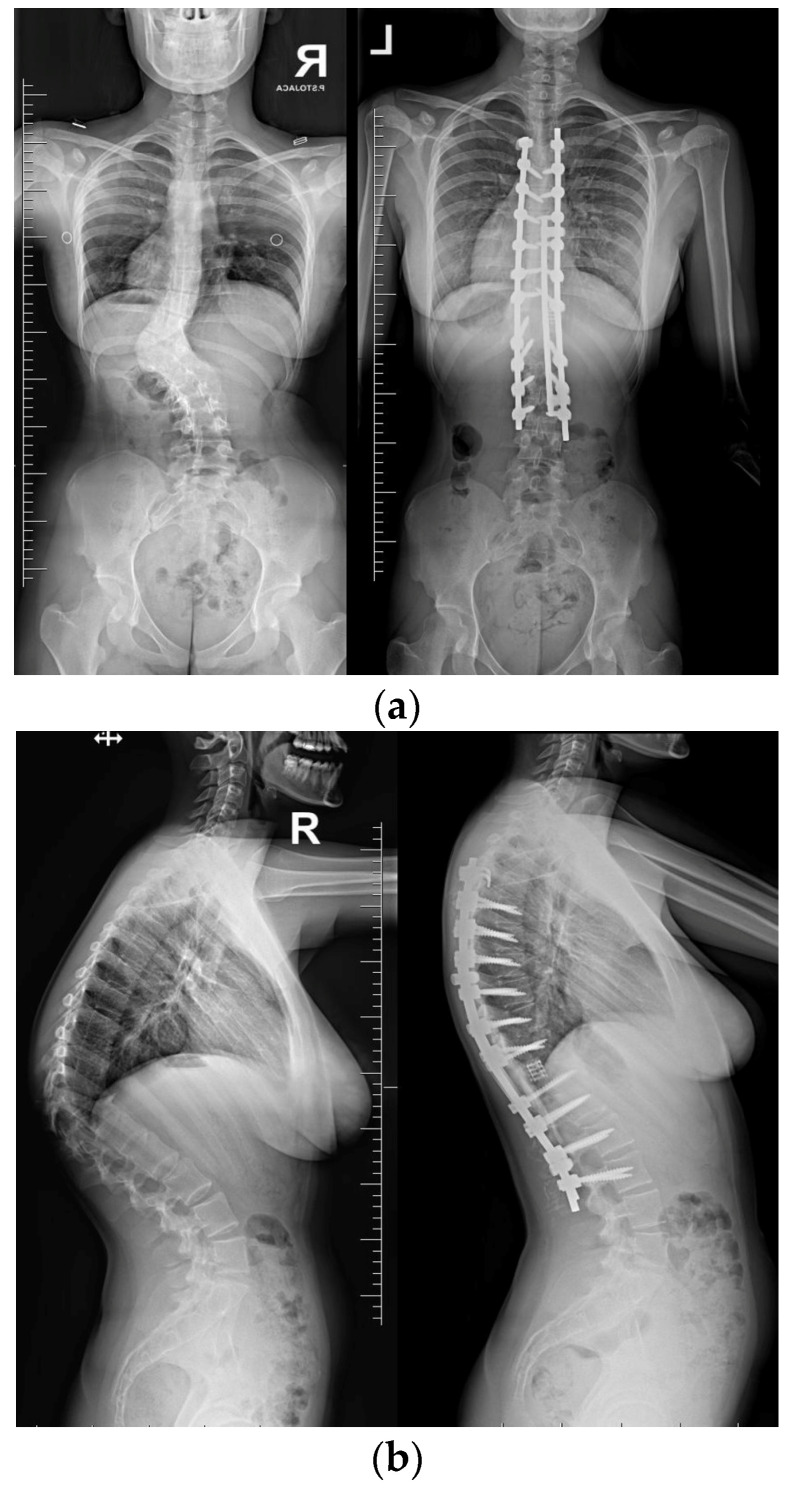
Standard standing AP (a) and lateral (b) X-rays of the 16-year-old female after undergoing surgical treatment. These X-rays were performed just after surgery.
